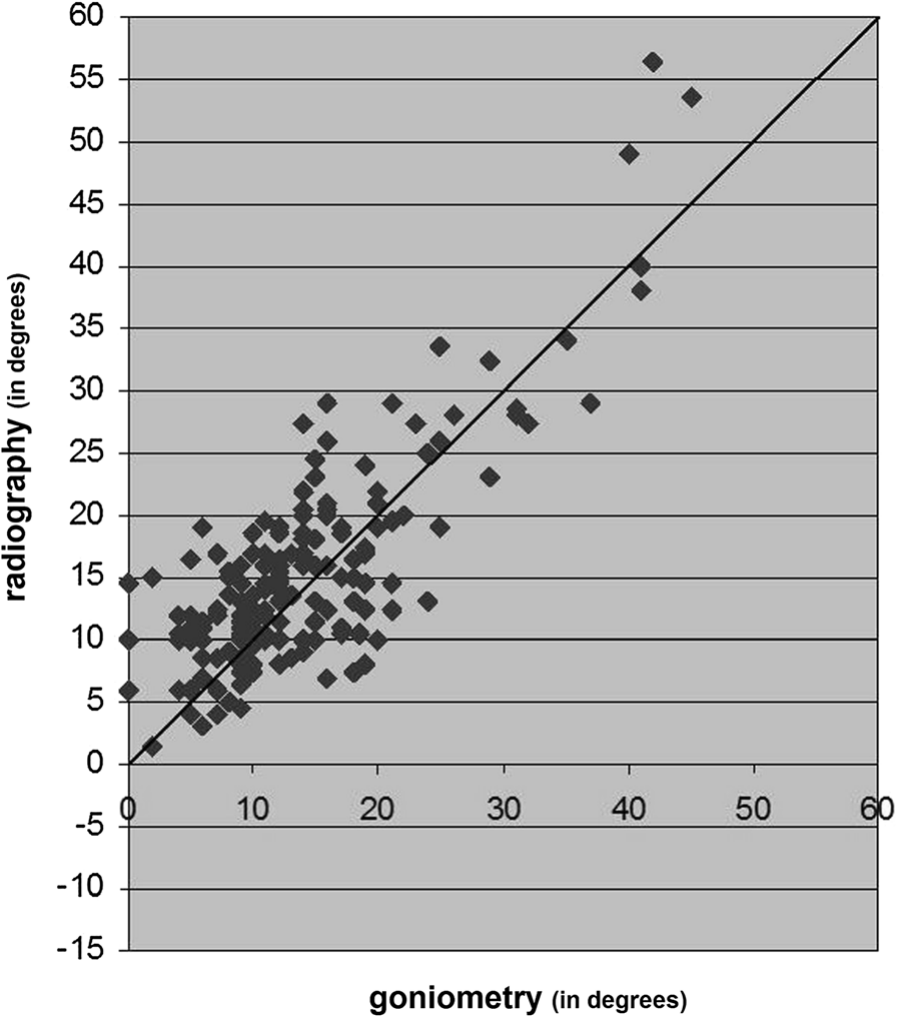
The results of radiographic measurement of HVA compared with the results of clinical goniometry of HVA.
